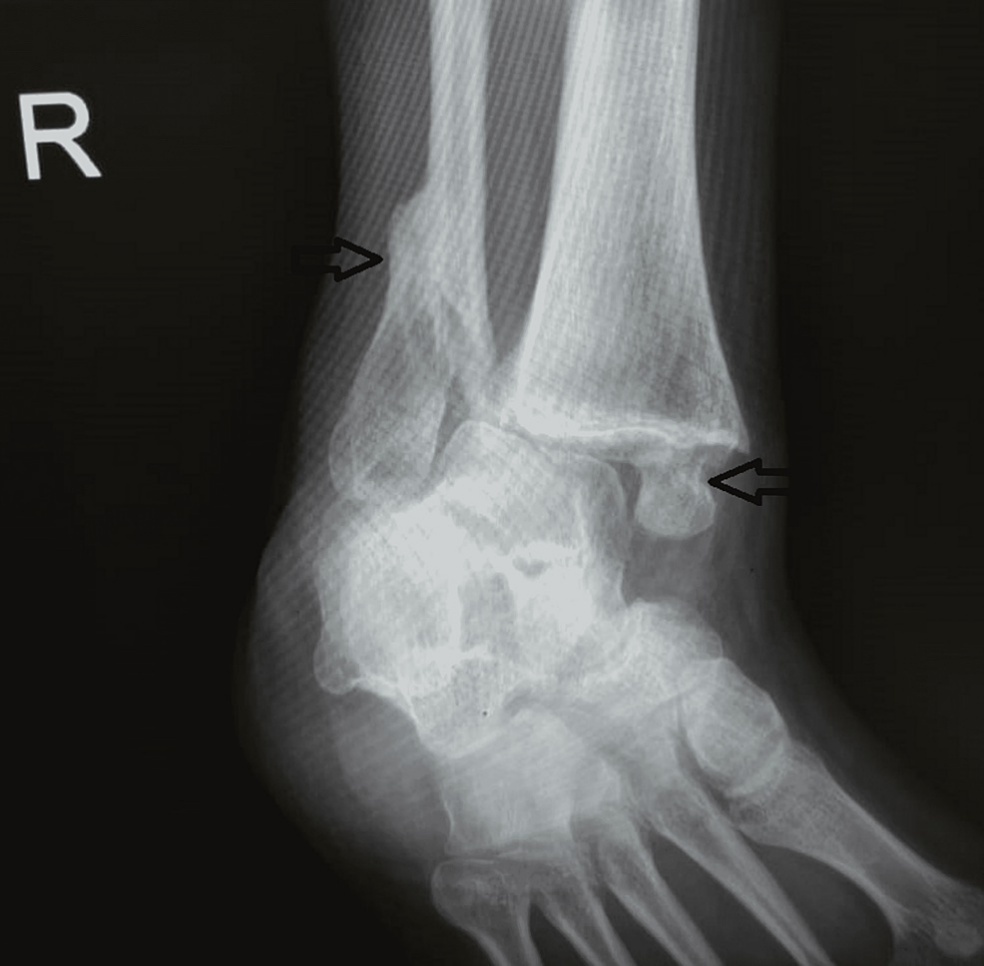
Preoperative plain radiograph anterior-posterior (AP) view showed the site of malunion
